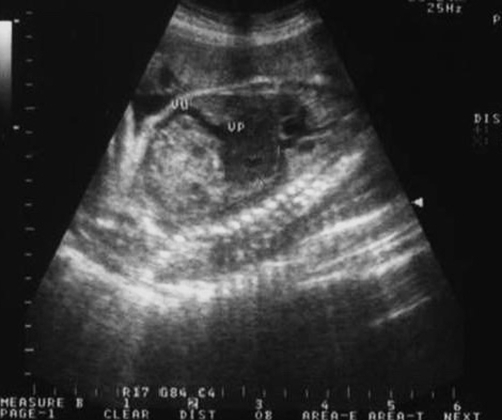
Hepatic lesion at prenatal ultrasound.
